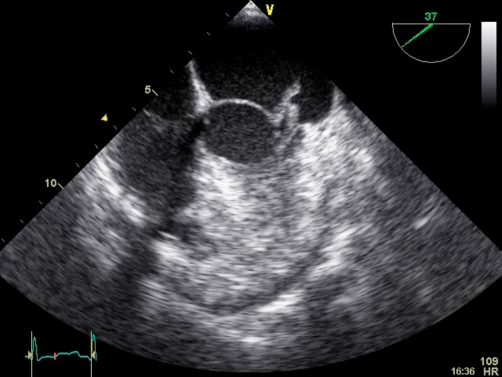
Echocardiogram showing mitral valve vegetation.
